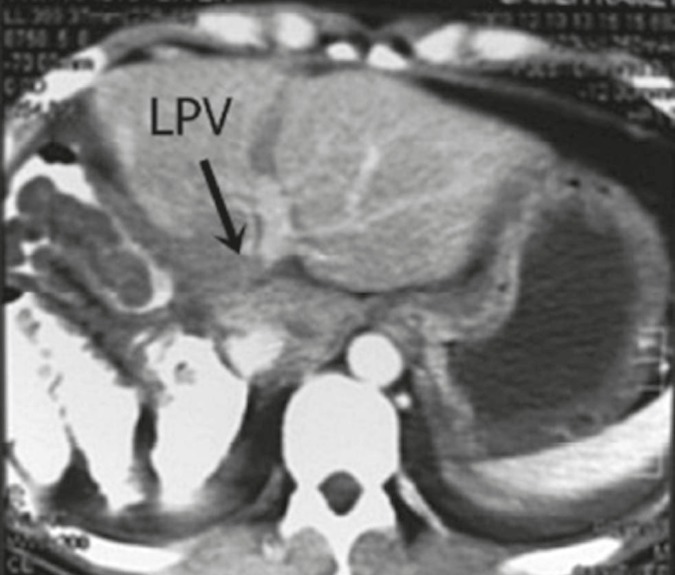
Triphasic CT portal phase showing a thrombus in the main and left portal vein (arrow) and opacified segmental branches.
